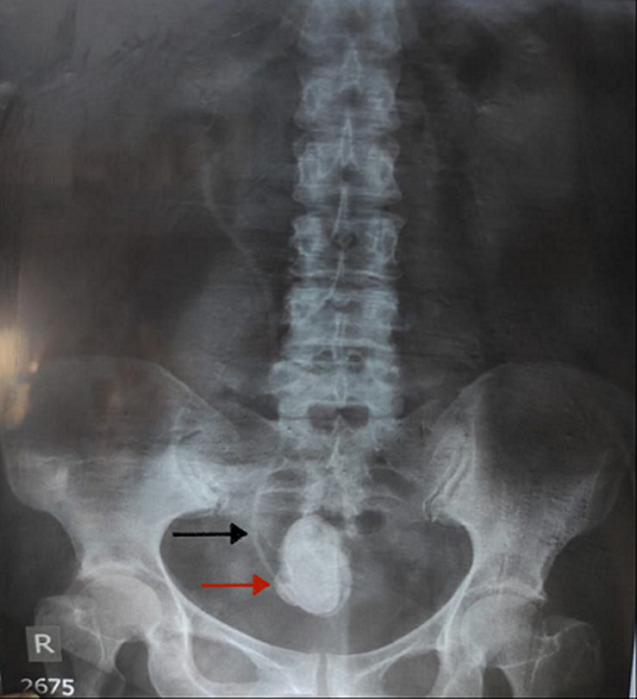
a kidney, ureter, and bladder X-ray showed a radiopaque image in the pelvis with a size of 45 mm x 30 mm (red arrow) and a stent image (black arrow) in the right paravertebral space of the pelvic cavity
